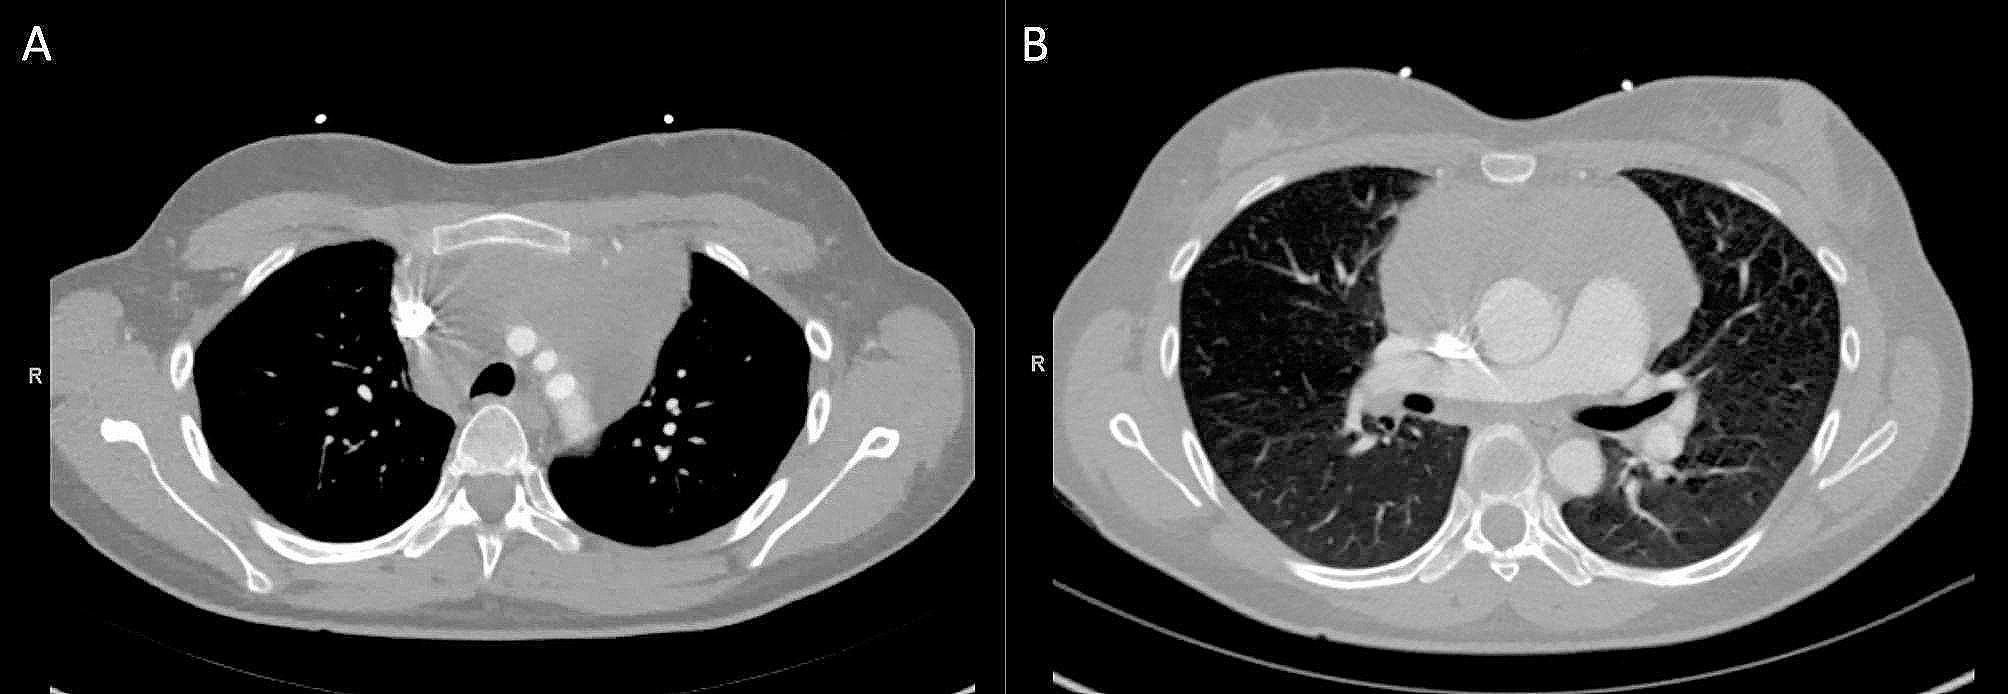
Posttreatment chest computed tomography (A, B) A heterogeneous 11 × 6 × 10 cm soft tissue mass within the anterior mediastinum. Decreased size and mass effect compared to pretreatment chest computed tomography
